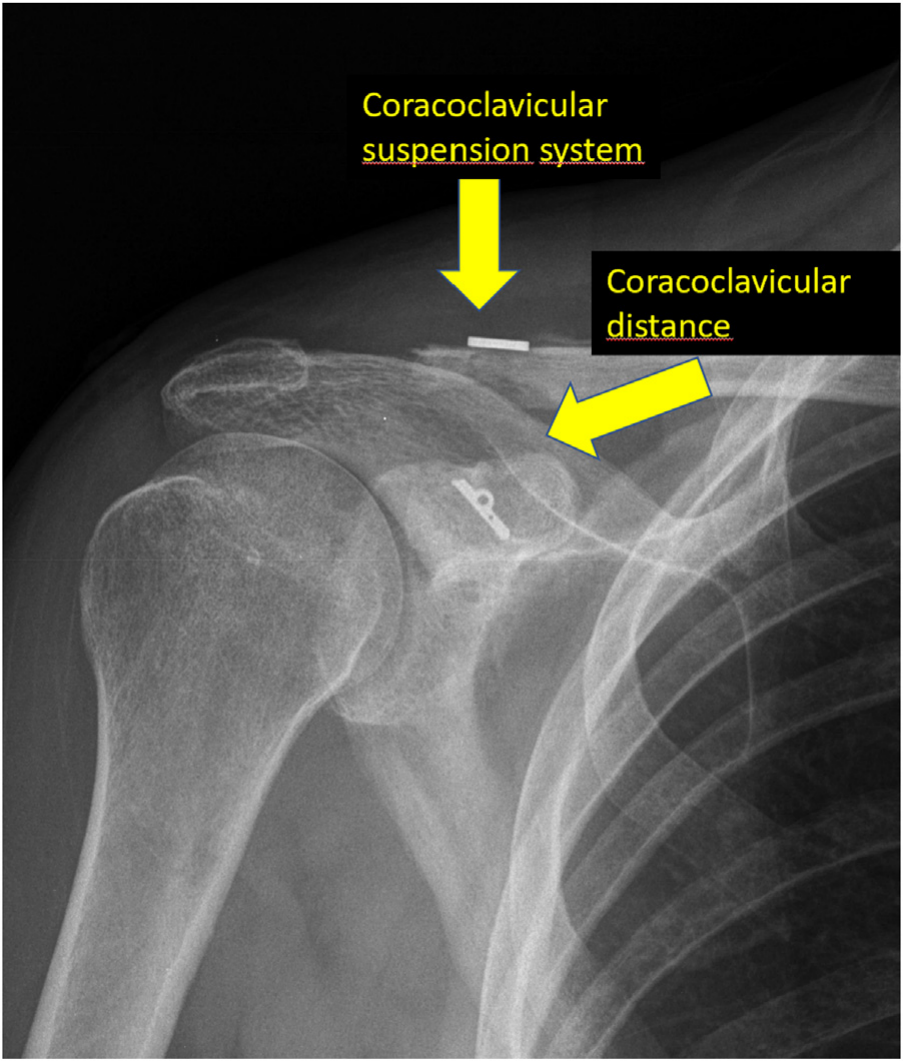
Postoperative control radiograph with decrease of coracoclavicular distance and increase of acromioclavicular distance due to the resection of 4 to 5 mm of the distal clavicle.
